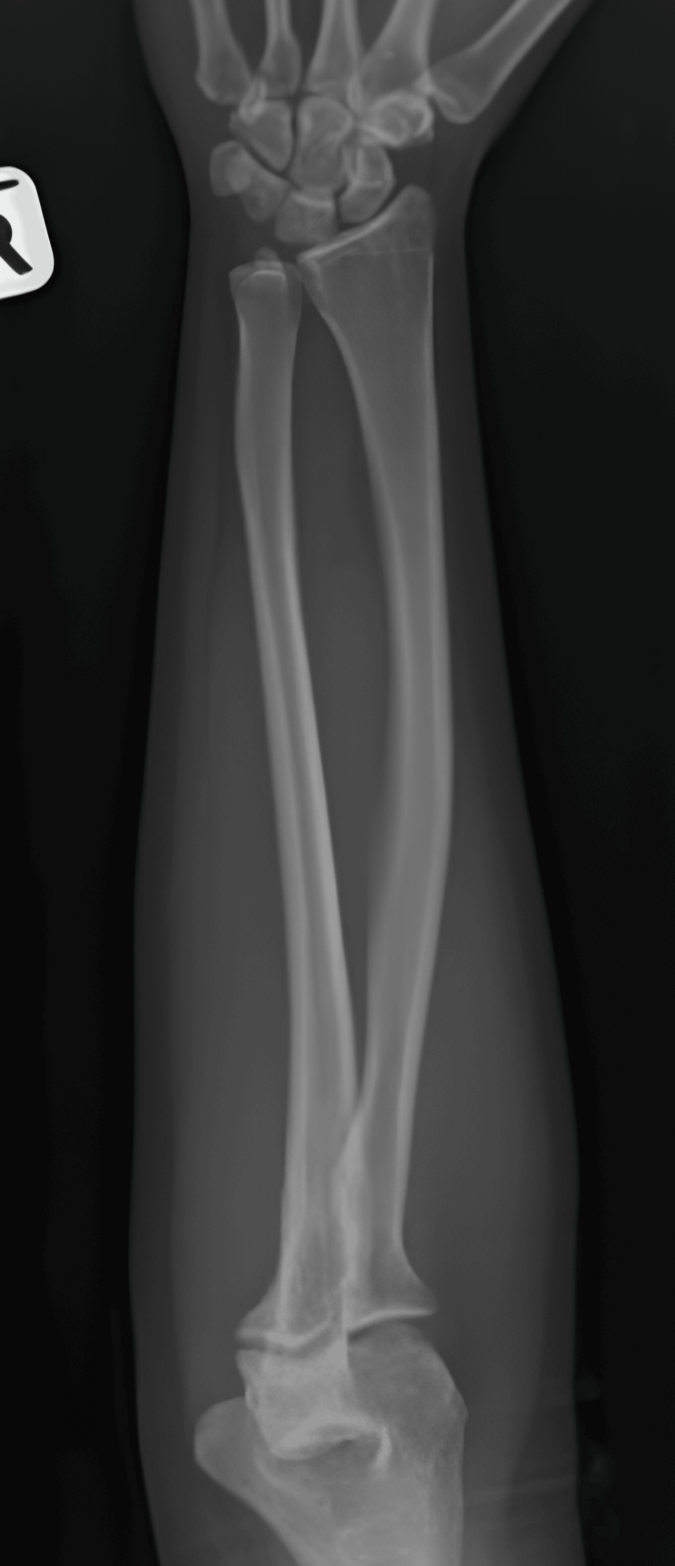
Plain radiograph of an anteroposterior view of the right forearm The radiograph of the right forearm shows no bony lesions or abnormal calcifications, with unremarkable surrounding soft tissues.
